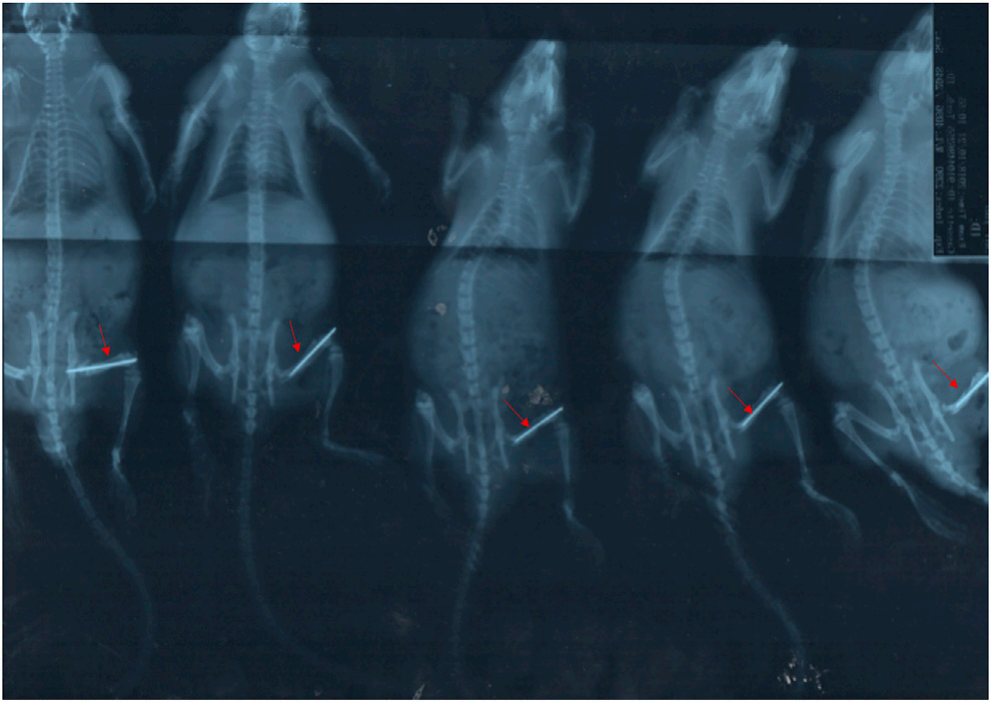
X-ray of the closed femoral shaft fracture Model.
